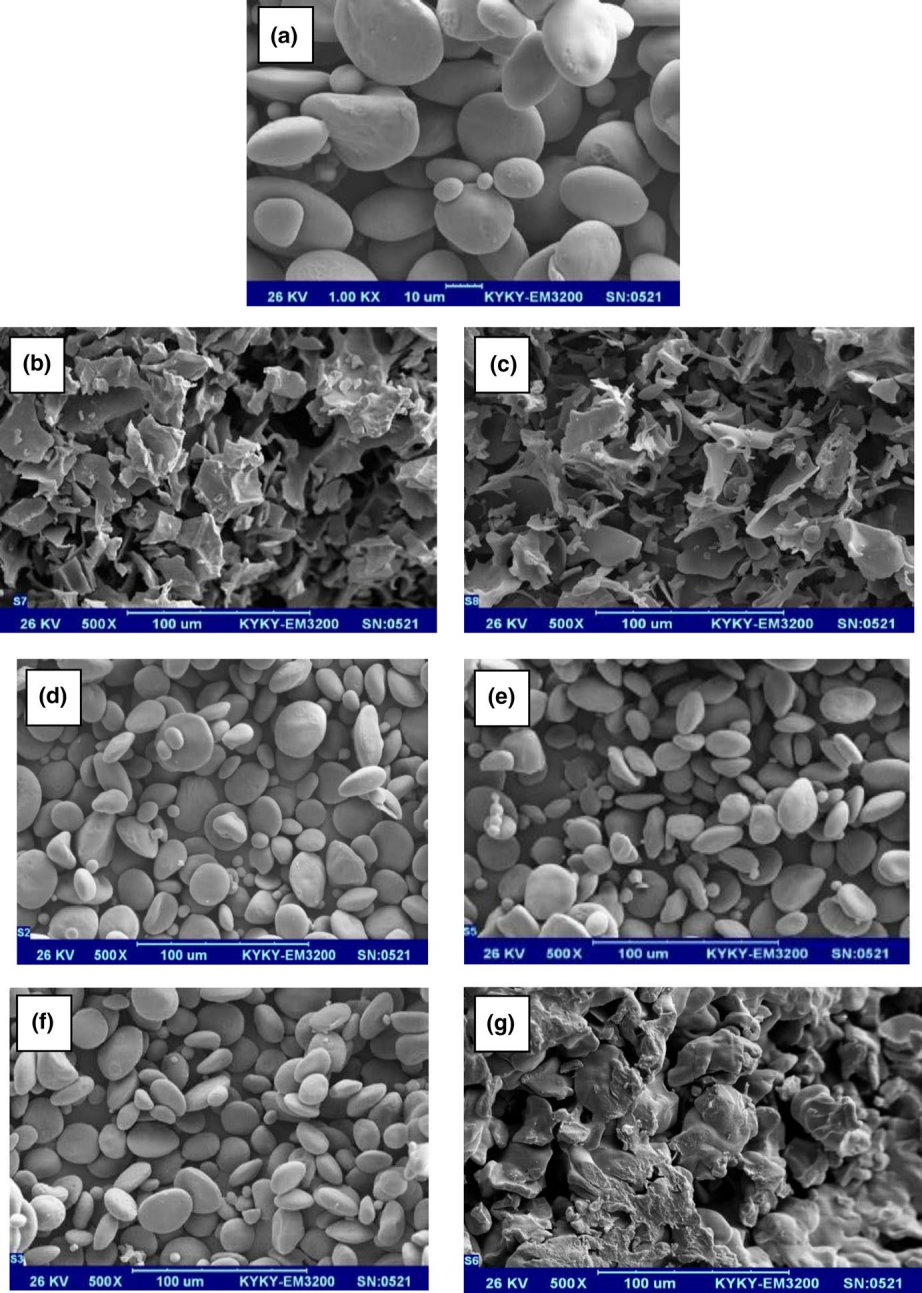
SEM images for the wheat starch‐sonicated samples. (a) control, untreated sample; (b) ultrasound probe, 200 W–15 min; (c) ultrasound probe, 200 W–30 min; (d) ultrasound probe, 400 W–15 min; (e) ultrasound probe, 400 W–30 min; (f) ultrasound bath, 690 W–15 min; (g) ultrasound bath, 690 W–30 min
